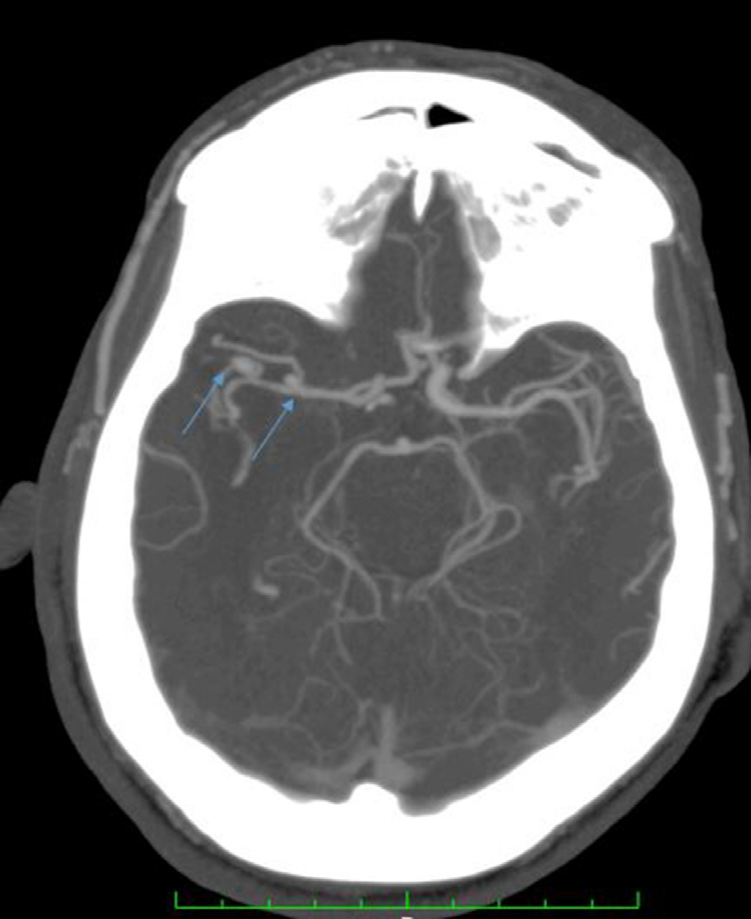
Axial section of cerebral CT scan in MIP (maximum intensity projection) reconstruction showing 2 aneurysms in the right M1 branch (arrows).
